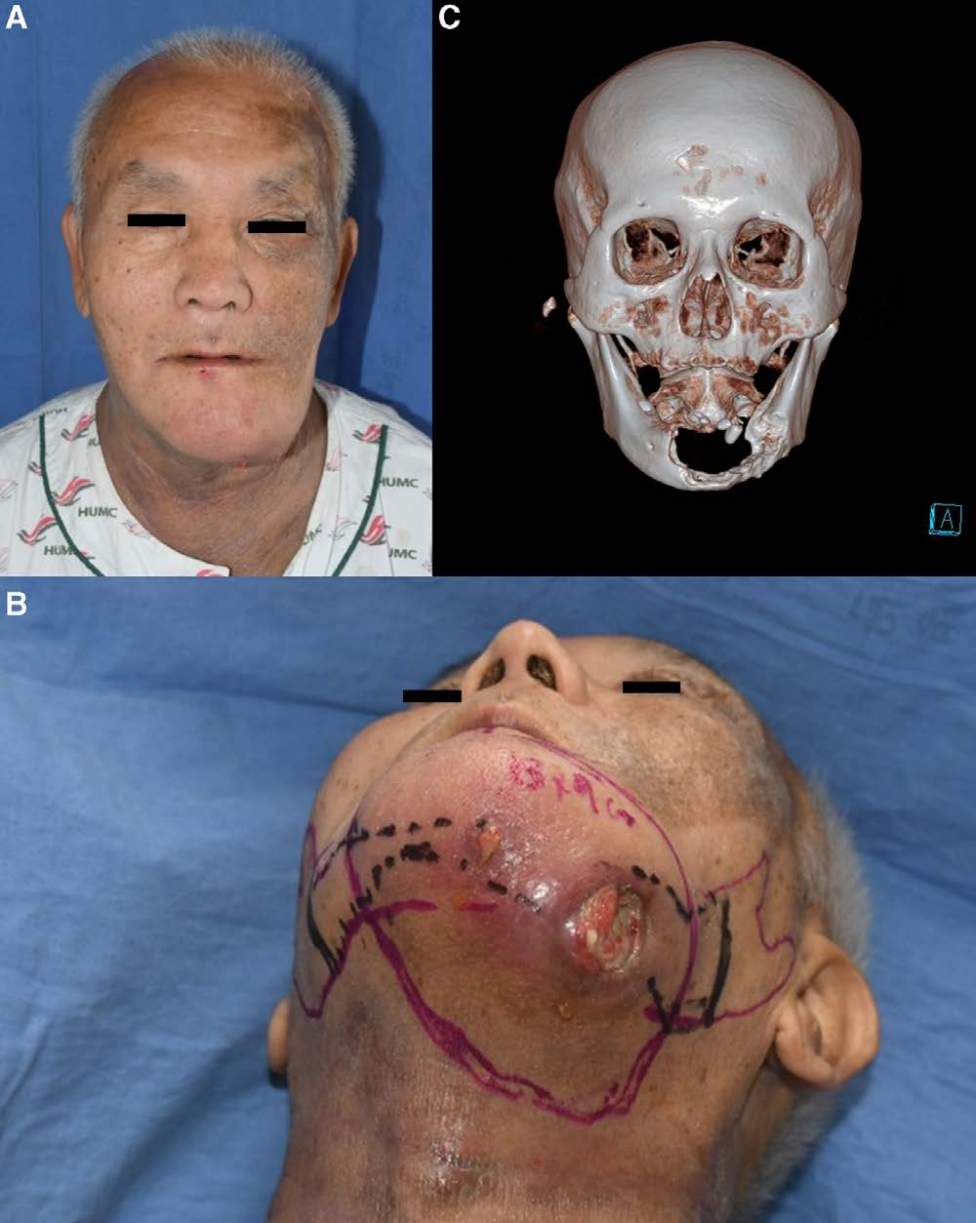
Preoperative clinical photograph and computed tomography scan. (A) Front view. (B) The ulcerative mass on the left neck extended to the tongue, oral mucosa, and mandibular bone. (C) Mandibular bone destruction by invading squamous cell carcinoma.
